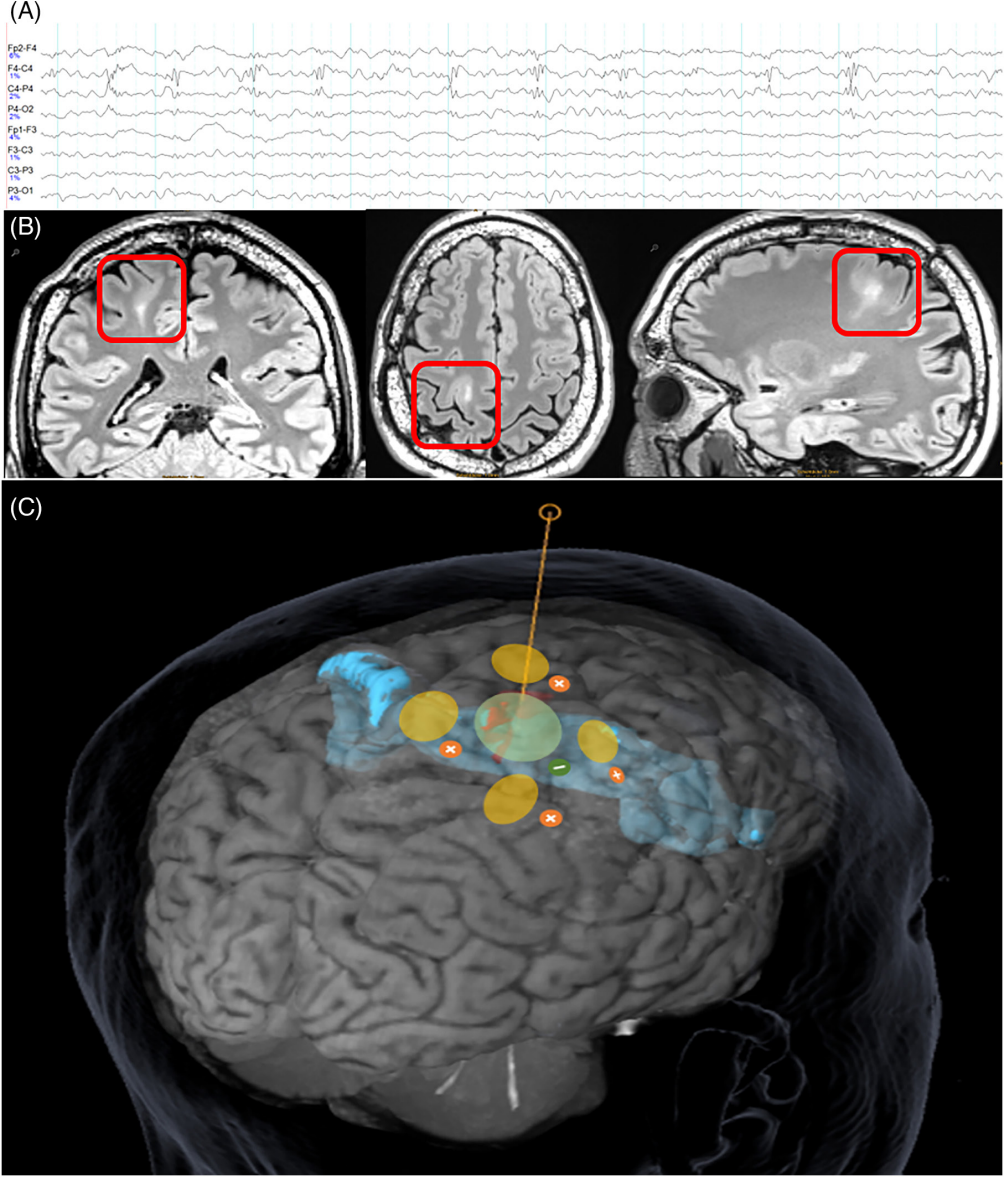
Electrode positioning based on delineation of the epileptic focus by EEG and MRI. A, Scalp EEG with frequent epileptic polyspike discharges in EEG over the right central region. B, MR Imaging of a right premotor suspected bottom‐of‐sulcus dysplasia with a typical tail sign (Siemens PRISMA 3T, 3D T2‐FLAIR‐MR images in coronal, axial, and sagittal direction). C, positioning of the electrode array with electrodes in green and yellow over the bottom‐of‐sulcus dysplasia (in red) and in relation to the primary motor cortex (blue segment).
